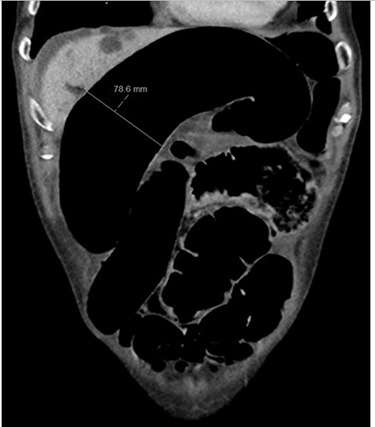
Case two: a coronal slice of a CT scan of the abdomen and pelvis with intravenous contrast showing dilated large bowel loops; the radiology report suggested sigmoid volvulus with a centrally lying caecum.
